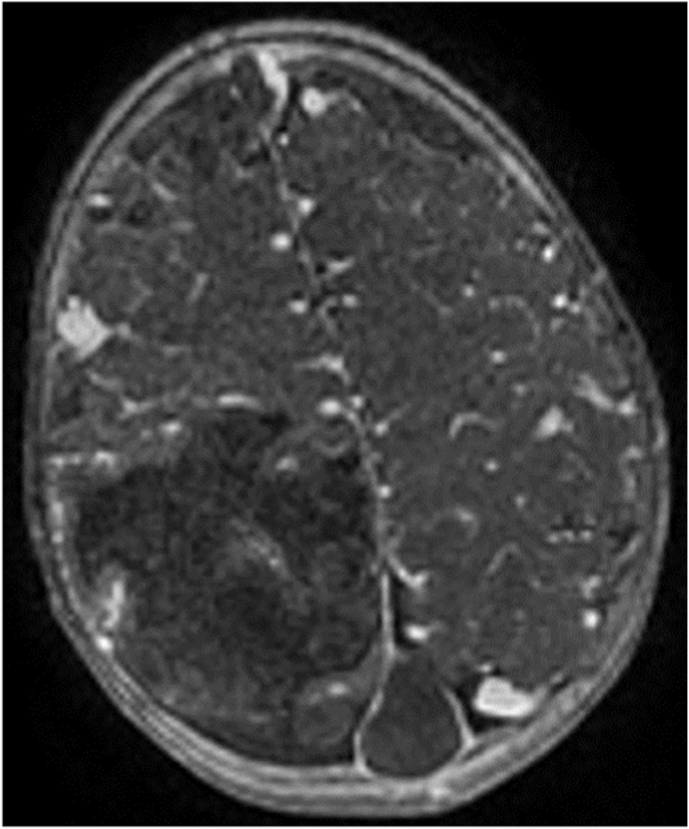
Lack of opacification of the dural venous sinuses in 3D FSPGR (fast spoiled gradient echo) MRI sequence.
